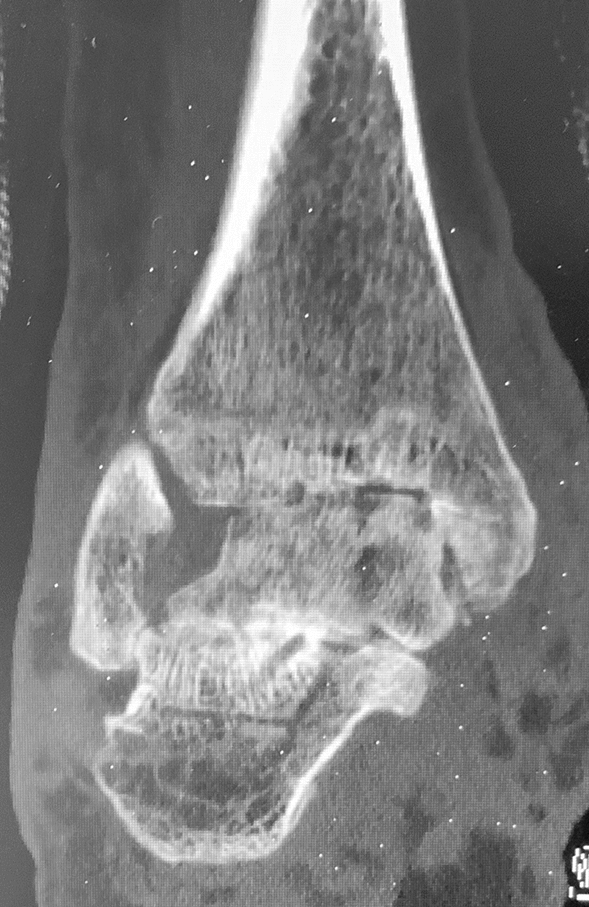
CT scan 14 weeks postoperatively shows fused TTC arthrodesis
